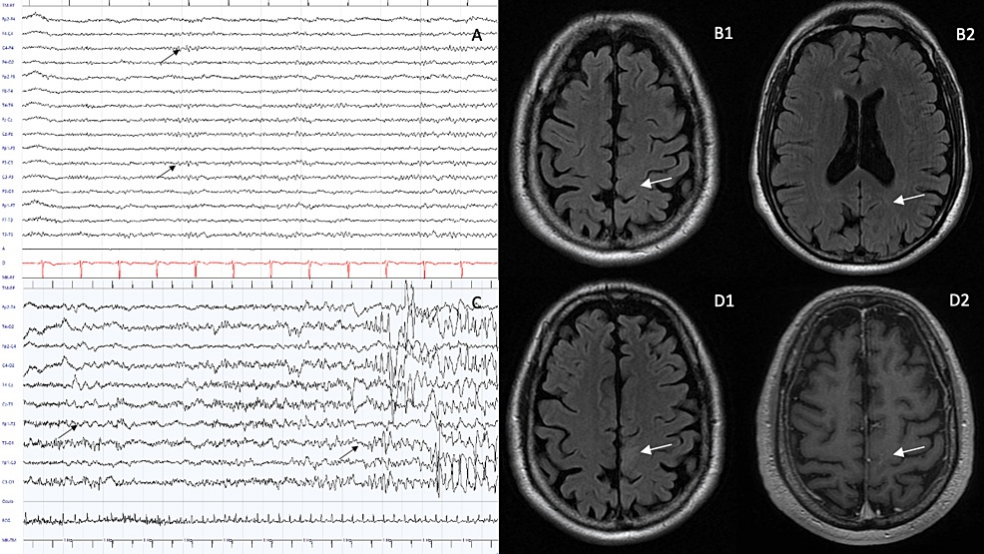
MRI and EEG findings EEG recording on hospital admission, showing only mild slowing in parasagittal chains (A); uncontrasted MRI FLAIR sequences, on day four after admission, showing normal findings in frontal mesial fields (B1, B2); EEG recording during a focal motor seizure with impaired awareness, showing diffuse slowing with left frontocentral slow and spiked activity with secondary generalization (C); MRI on day 11 after admission displaying a left frontomesial hyperintense lesion in T2 FLAIR sequences (D1) and mild contrast-enhancement (D2) MRI: magnetic resonance imaging; EEG: electroencephalography; FLAIR: fluid-attenuated inversion recovery
